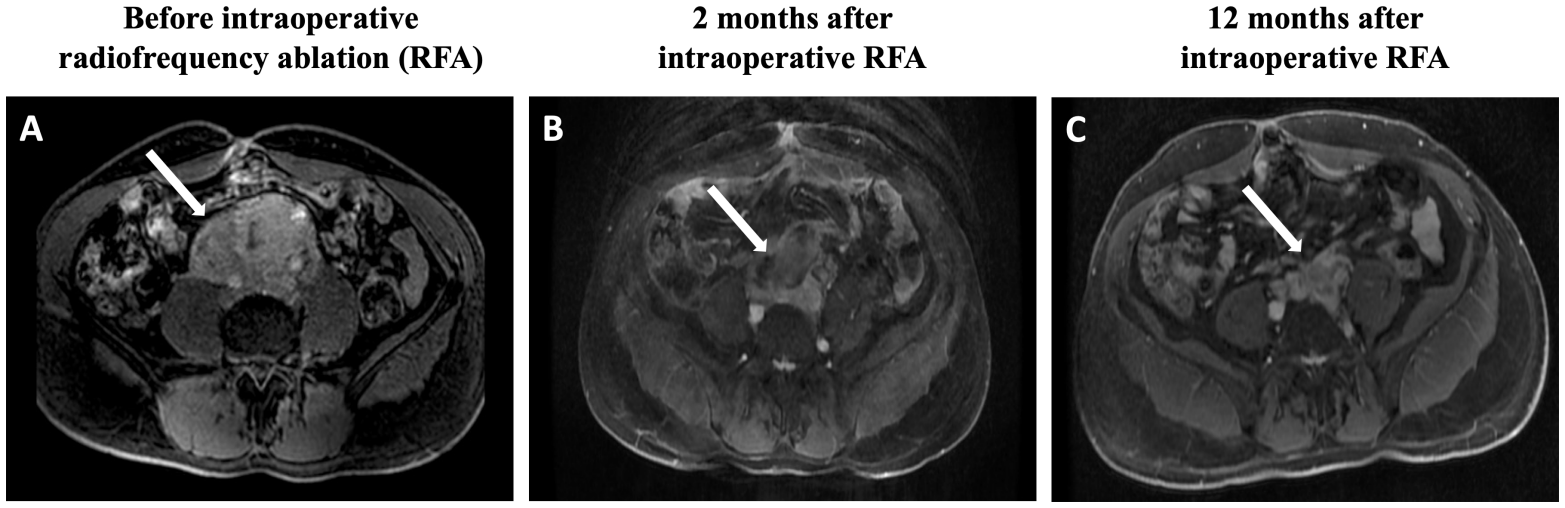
Magnetic resonance imaging findings: (A) Postgadolinium fat-saturation axial T1 weighted images reveal an extensive retroperitoneal mass, which measures 9.0 × 8.6 × 6.0 cm and involves the inferior portion of the vein inferior vena cava, inferior mesenteric artery, and infrarenal aorta. (B) The tumor size is reduced to 6.9 × 6.8 × 5.4 cm. (C) After 12 months of the RFA procedure, the tumor further shrinks to 7.2 × 4.8 × 4.1 cm. RFA, radiofrequency ablation.
